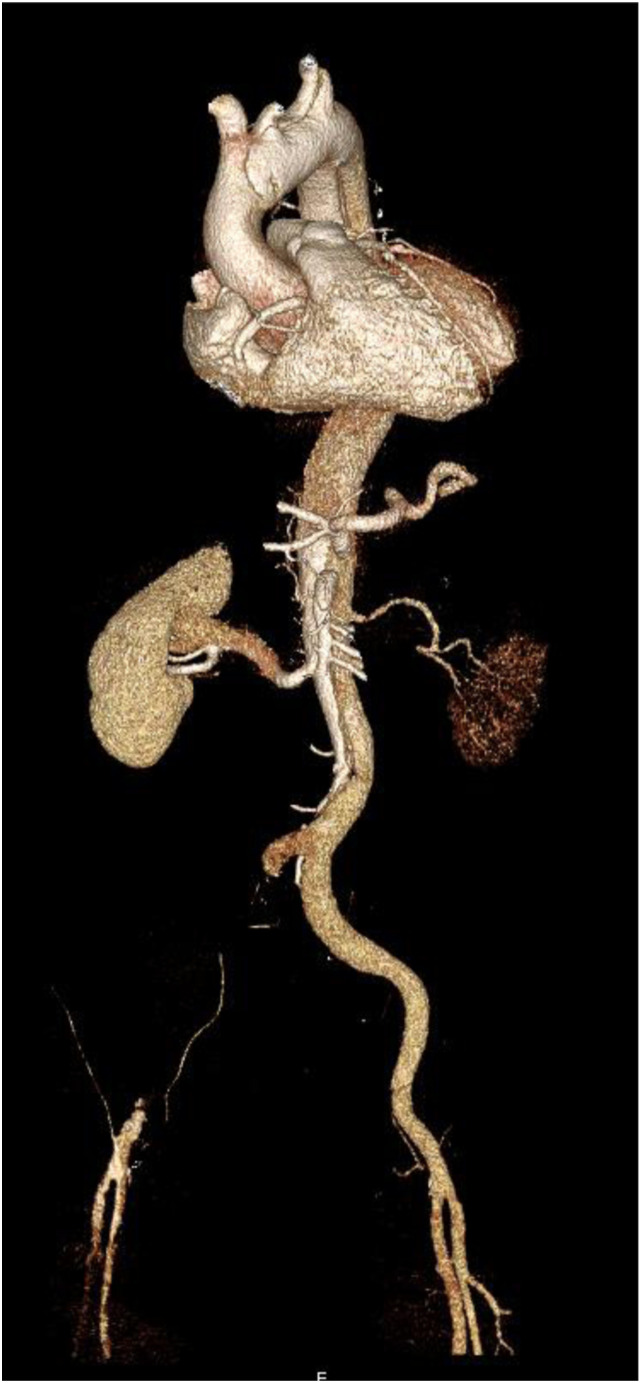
3D CTA reconstruction; ATAAD with malperfusion left renal artery and right common iliac artery.
